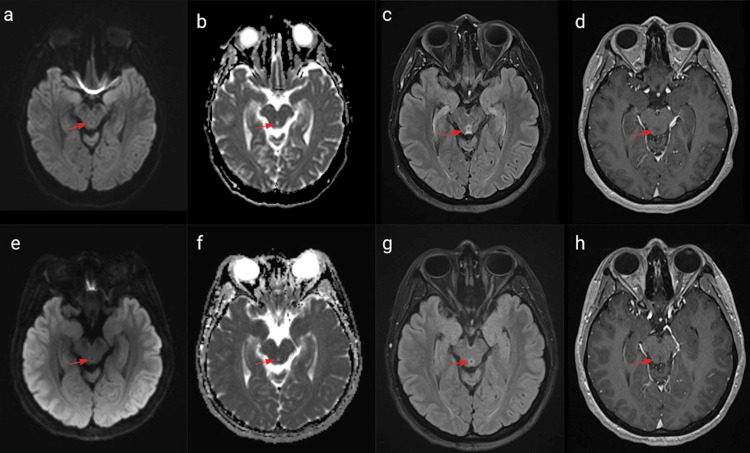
MRI brain comparison of the periaqueductal region on admission and four months later MRI brain comparison of the periaqueductal region on admission (the top row) and four months later (the bottom row): diffusion restriction (a, b) and hyperintensity in T2-weighted-Fluid-Attenuated Inversion Recovery (T2 FLAIR) sequence(c). Resolving signal abnormalities with minimal diffusion restriction (e, f) and trace residual T2/FLAIR signal (g) on the periaqueductal region. T1-weighted images are normal (d, h).
